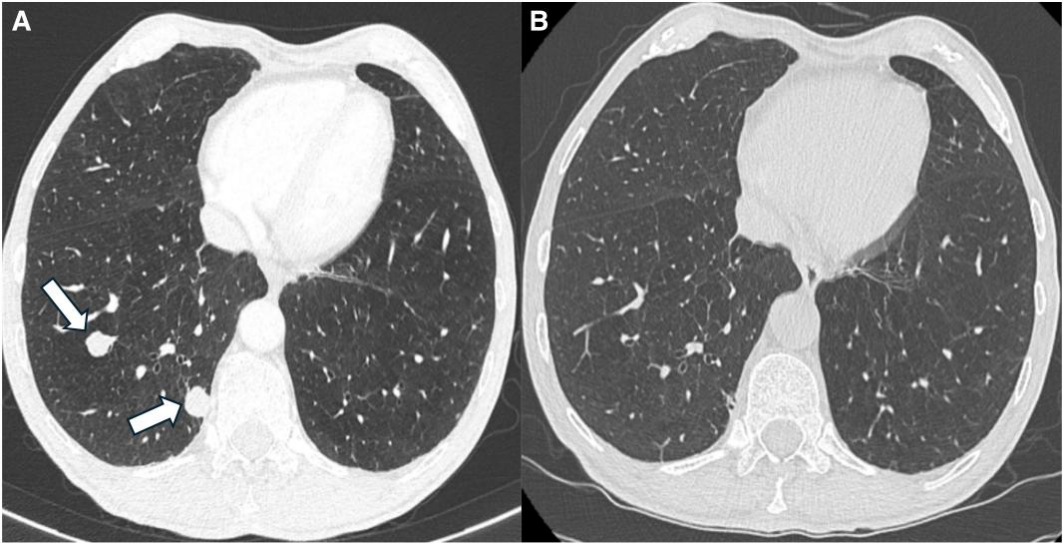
Pre-and posttreatment CT of the lung of the patient. Axial CT image at the initial presentation (A) demonstrates 2 well-circumscribed pulmonary nodules in the right lower lobe (arrows) consistent with metastases. Corresponding CT image at the same level (B), 6 months posttreatment, shows complete resolution of the metastatic nodules. Abbreviations: CT, computed tomography.
